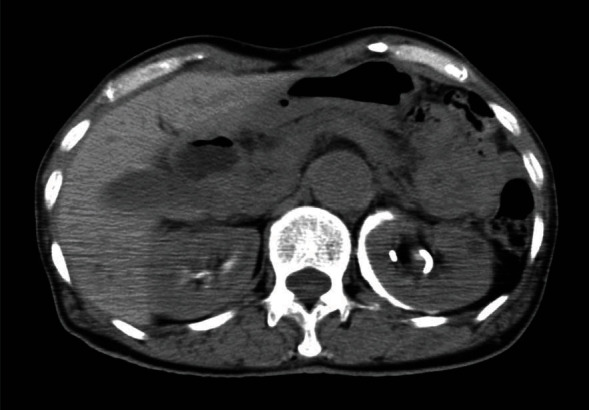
CT shows contrast leakage in the retroperitoneal space.
